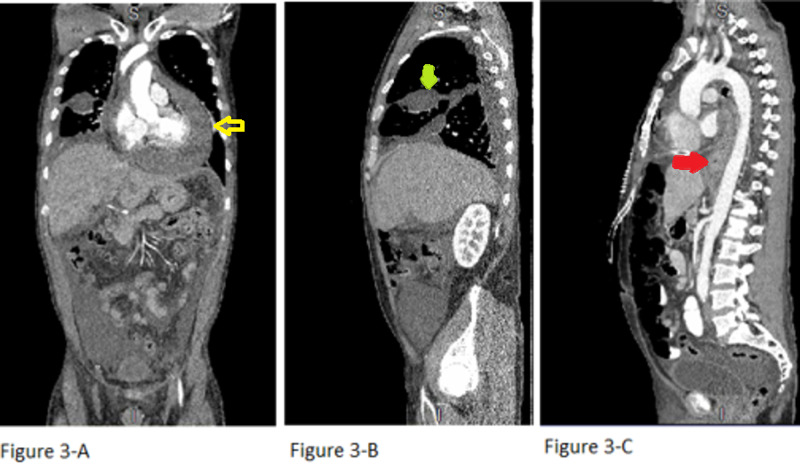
Abdomen and chest CT with contrast Large circumferential pericardial effusion measuring up to 4.3 cm, bilateral pleural effusions larger on the right with associated compressive and dependent atelectasis (3-A) with pleural fluid accumulation within the middle and lower fissure (3-A and 3-B), esophageal wall thickening (3-C), and mediastinal and hilar adenopathy CT: computed tomography
